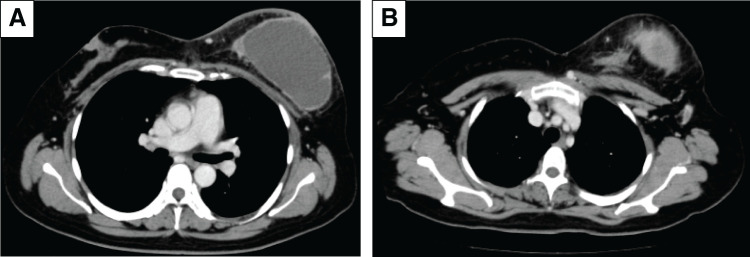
Contrast-enhanced CT captured on cycle 2 day 22 (A: left breast tumor with skin edema; B: shrunken axillary lymph node). These findings suggest inflammatory changes in the breast tumor.
